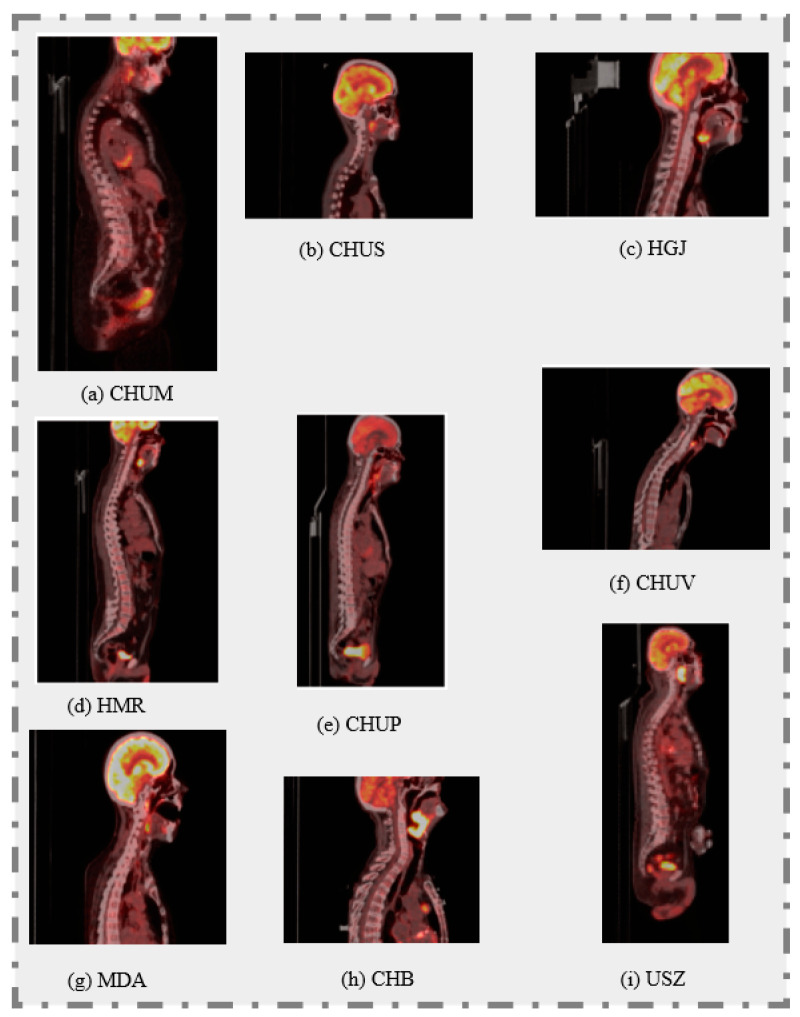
2D sagittal slices of fused PET/CT images from each of the nine participating centers.
